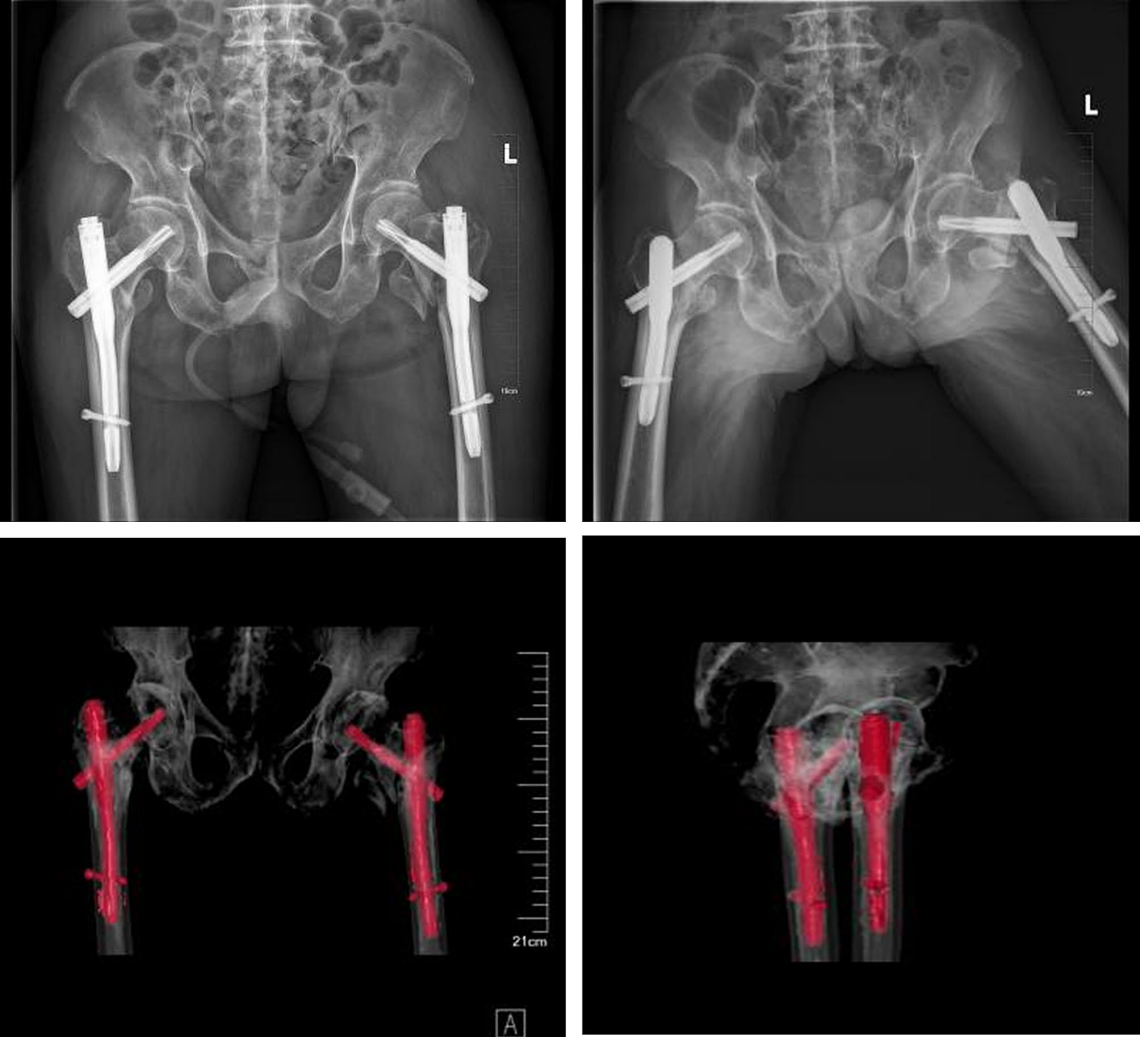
Postoperative X-ray and CT three-dimensional volume rendering (VR) indicated satisfactory position of PFNA without helical blade cuts
